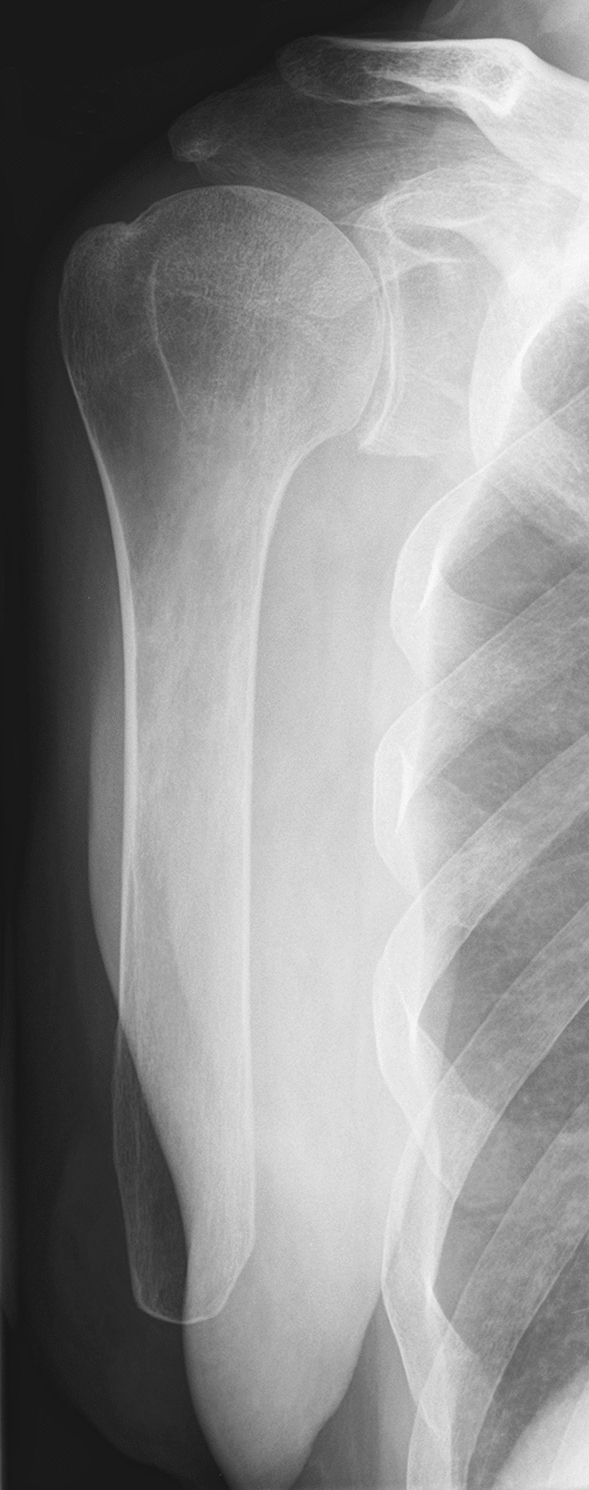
Radiographic example of a upper limb amputation (transhumeral) following a motor vehicle accident
